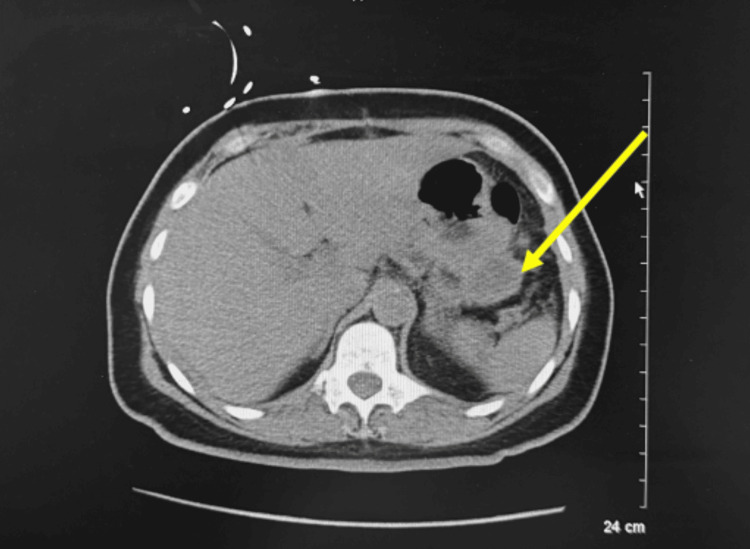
CT Abd/Pelvis with IV contrast on POD 18 showing a significant decrease in size measuring 2.1 x 3.7cm (as represented by the yellow arrow).
